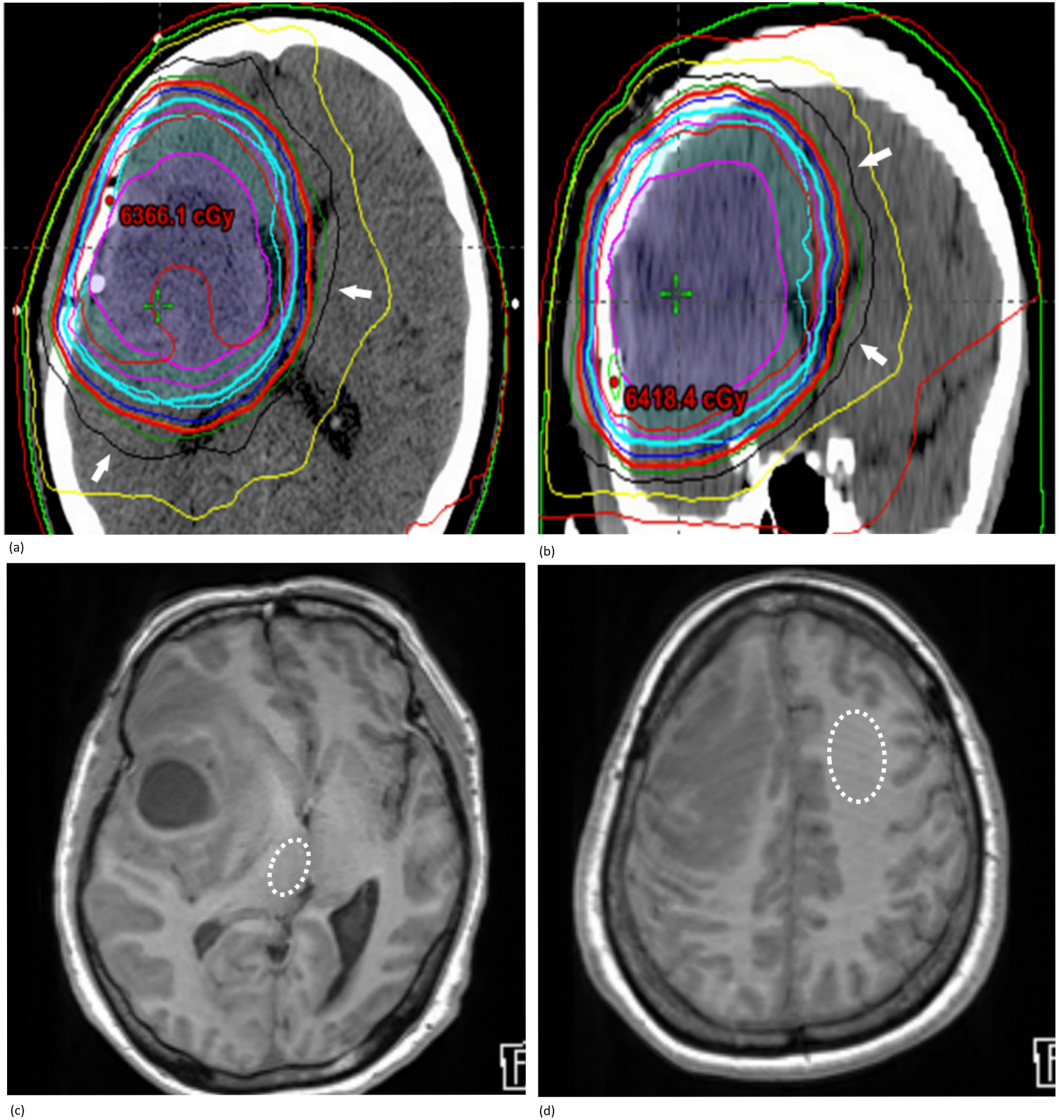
Definition of Area_H_ and Area_L_. A 58-year-old female patient underwent radiotherapy for the treatment of GBM located in the right frontal lobe. The radiotherapy plan map was obtained from the electronic medical records system (EMR) and was composed of representative (a) axial, (b) coronal, and sagittal (not presented) CT images (note; arrows represent the iso-dose line of 3600 cGy). In this patient, (c) the right thalamus (dashed circle) was designated as a Area_H_, whereas (d) the left frontal white matter (dashed circle) was considered a Area_L_ (presented images: pre-contrast T1WI).
